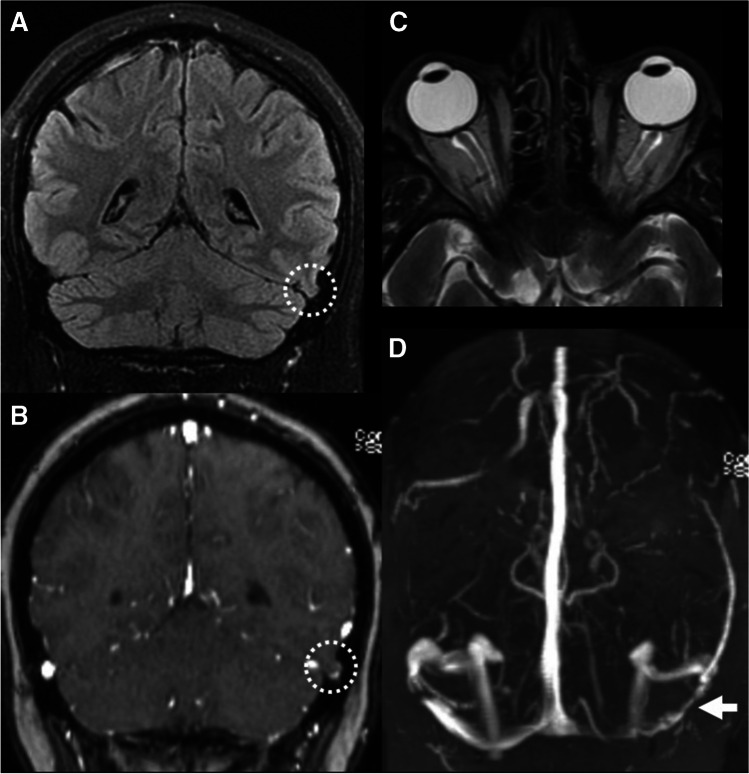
MR imaging example of IIH in the setting of a left transverse BHAG (patient 10 with right-sided PT). A, B Coronal T2 FLAIR and T1 post-contrast MR images show a small BHAG of the left posterior temporal lobe into the transverse sinus (dashed circle). C Axial T2 MR image shows flattening of the posterior globes and protrusion of the optic nerve heads as well as prominent optic nerve sheath subarachnoid space, findings suggestive of elevated intracranial pressure. D MR venogram shows bilateral transverse sinus stenoses, most pronounced on the left side at site of the BHAG (white arrow)
